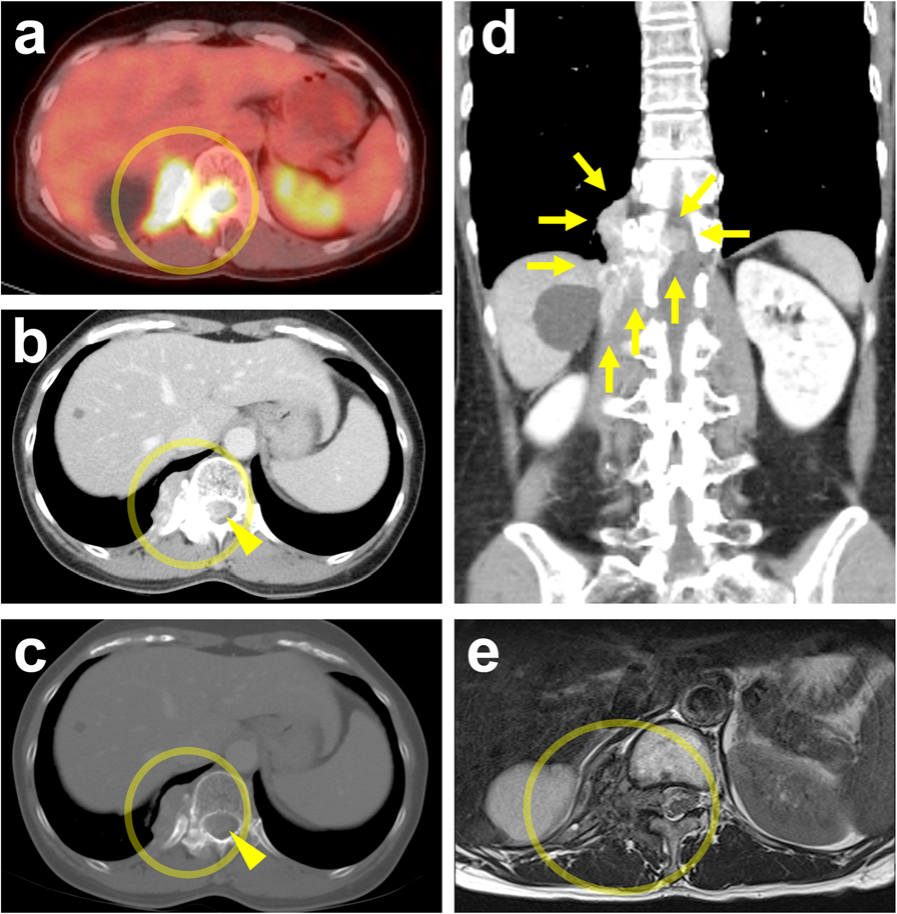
Imaging of the paraspinal lesion. a Positron emission tomography-CT fusion image demonstrating high fluorodeoxyglucose uptake (SUVmax 6.6). b and c Axial CT image showing a mixed osteosclerotic and osteolytic mass in the right arch of the Th12 vertebra (circled) on soft tissue (b) and bone (c) windows, protruding into the spinal canal (arrow heads). d Coronal reconstruction CT image revealing a bone metastasis infiltrating into surrounding soft tissue with peritoneal penetration through the diaphragm. e T2-weighted MRI image showing slightly high signal intensity in the same location (circled)
